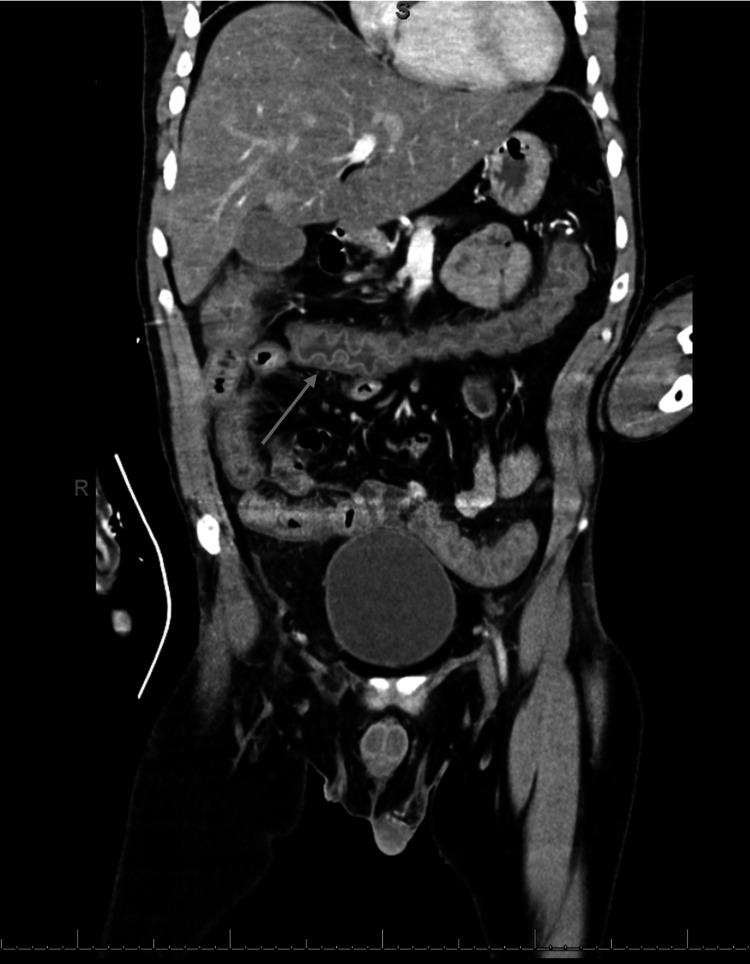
CT abdomen and pelvis with contrast, coronal plane showing diffuse colonic wall thickening
